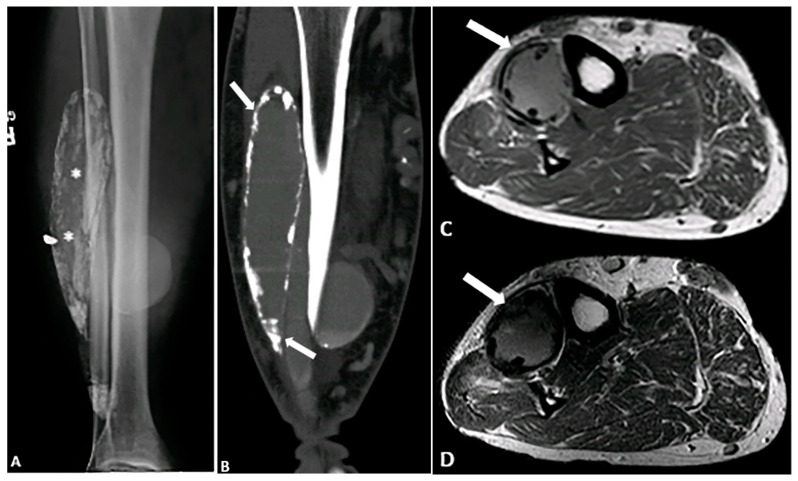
A 71-year-old male with a remote history of traumatic injury to the femoral artery and multiple fasciotomies. (A,B) Radiograph and CT demonstrate a calcified fusiform mass within the anterior compartment of the lower leg, with multiple foci of calcification within the mass and in its periphery (* and arrow). (C,D) Axial T1-weighted (C) and T2-weighted SPAIR (D) images reveal an intermediate signal intensity mass (arrows) with peripheral susceptibility artifacts due to calcium deposition. These findings are compatible with calcific myonecrosis. The image was obtained from the University of Washington Medical Imaging database with the required permissions.
